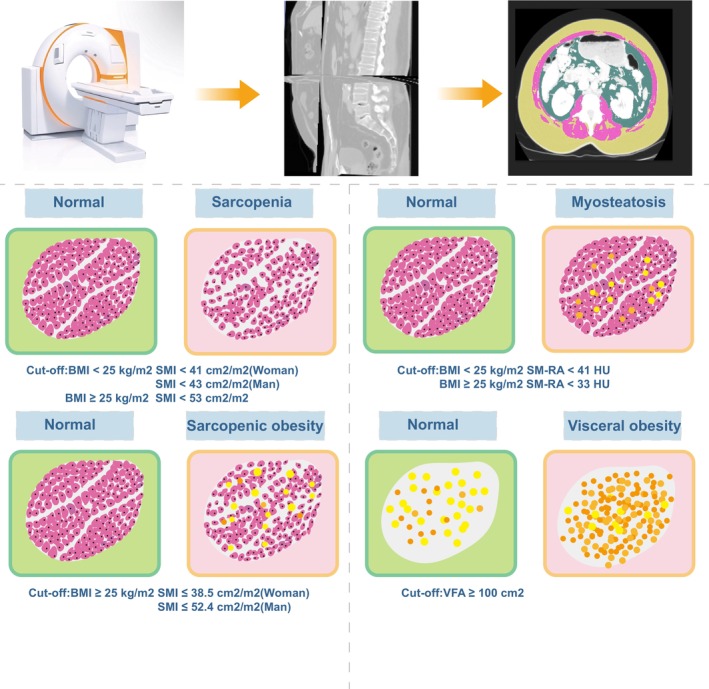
Post‐segmentation analysis of preoperative third lumbar spine CT in patients with perihilar cholangiocarcinoma for the identification of sarcopenia, myosteatosis, visceral obesity, and sarcopenic obesity. The corresponding regions were defined using the following attenuation values: Skeletal muscle region (purple), −29‐150 HU; subcutaneous fat region (yellow), −190 to −30 HU; visceral fat region (dark green), −150 to −50 HU. BMI, body mass index; VFA, visceral fat area.
